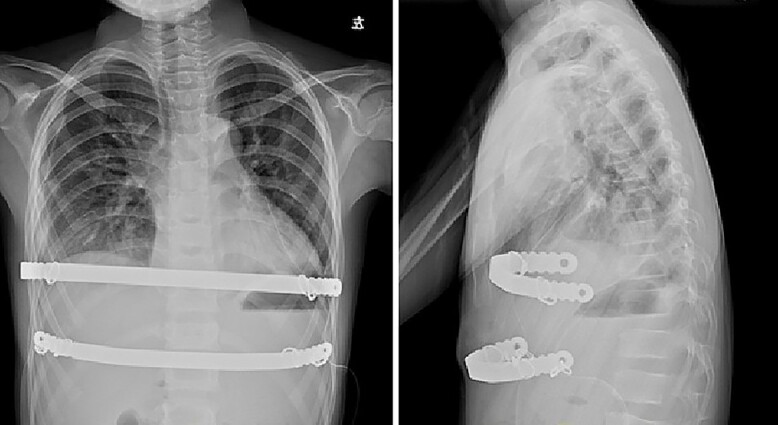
Chest X-ray examination after reoperation.
